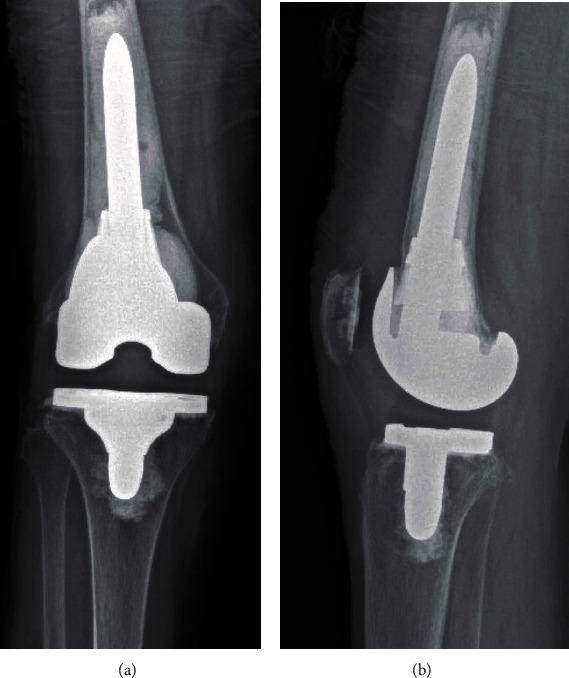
Right knee: (a) AP and (b) lateral radiographs at one-year follow-up.
